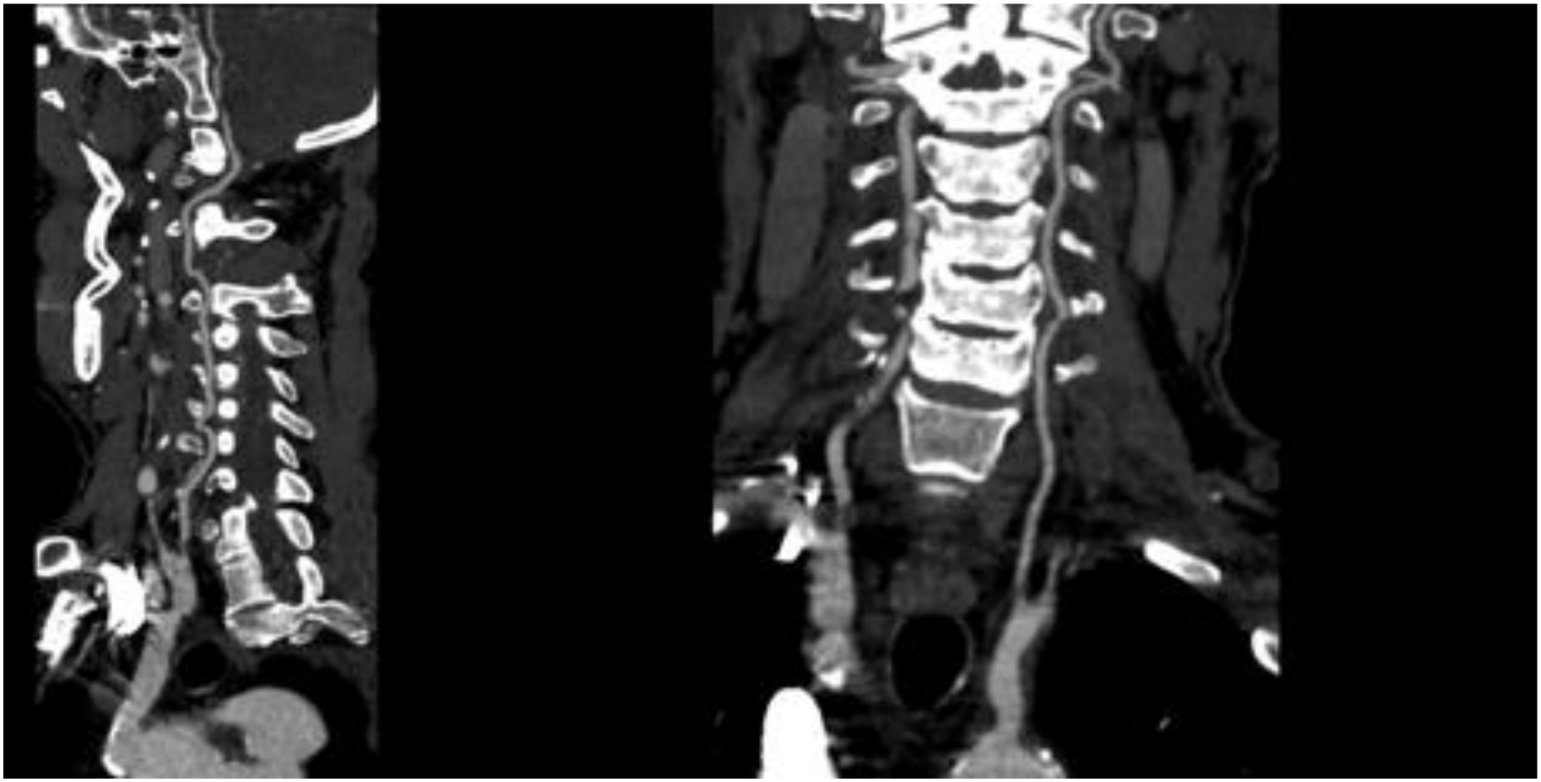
CTA of the cerebral arteries.
